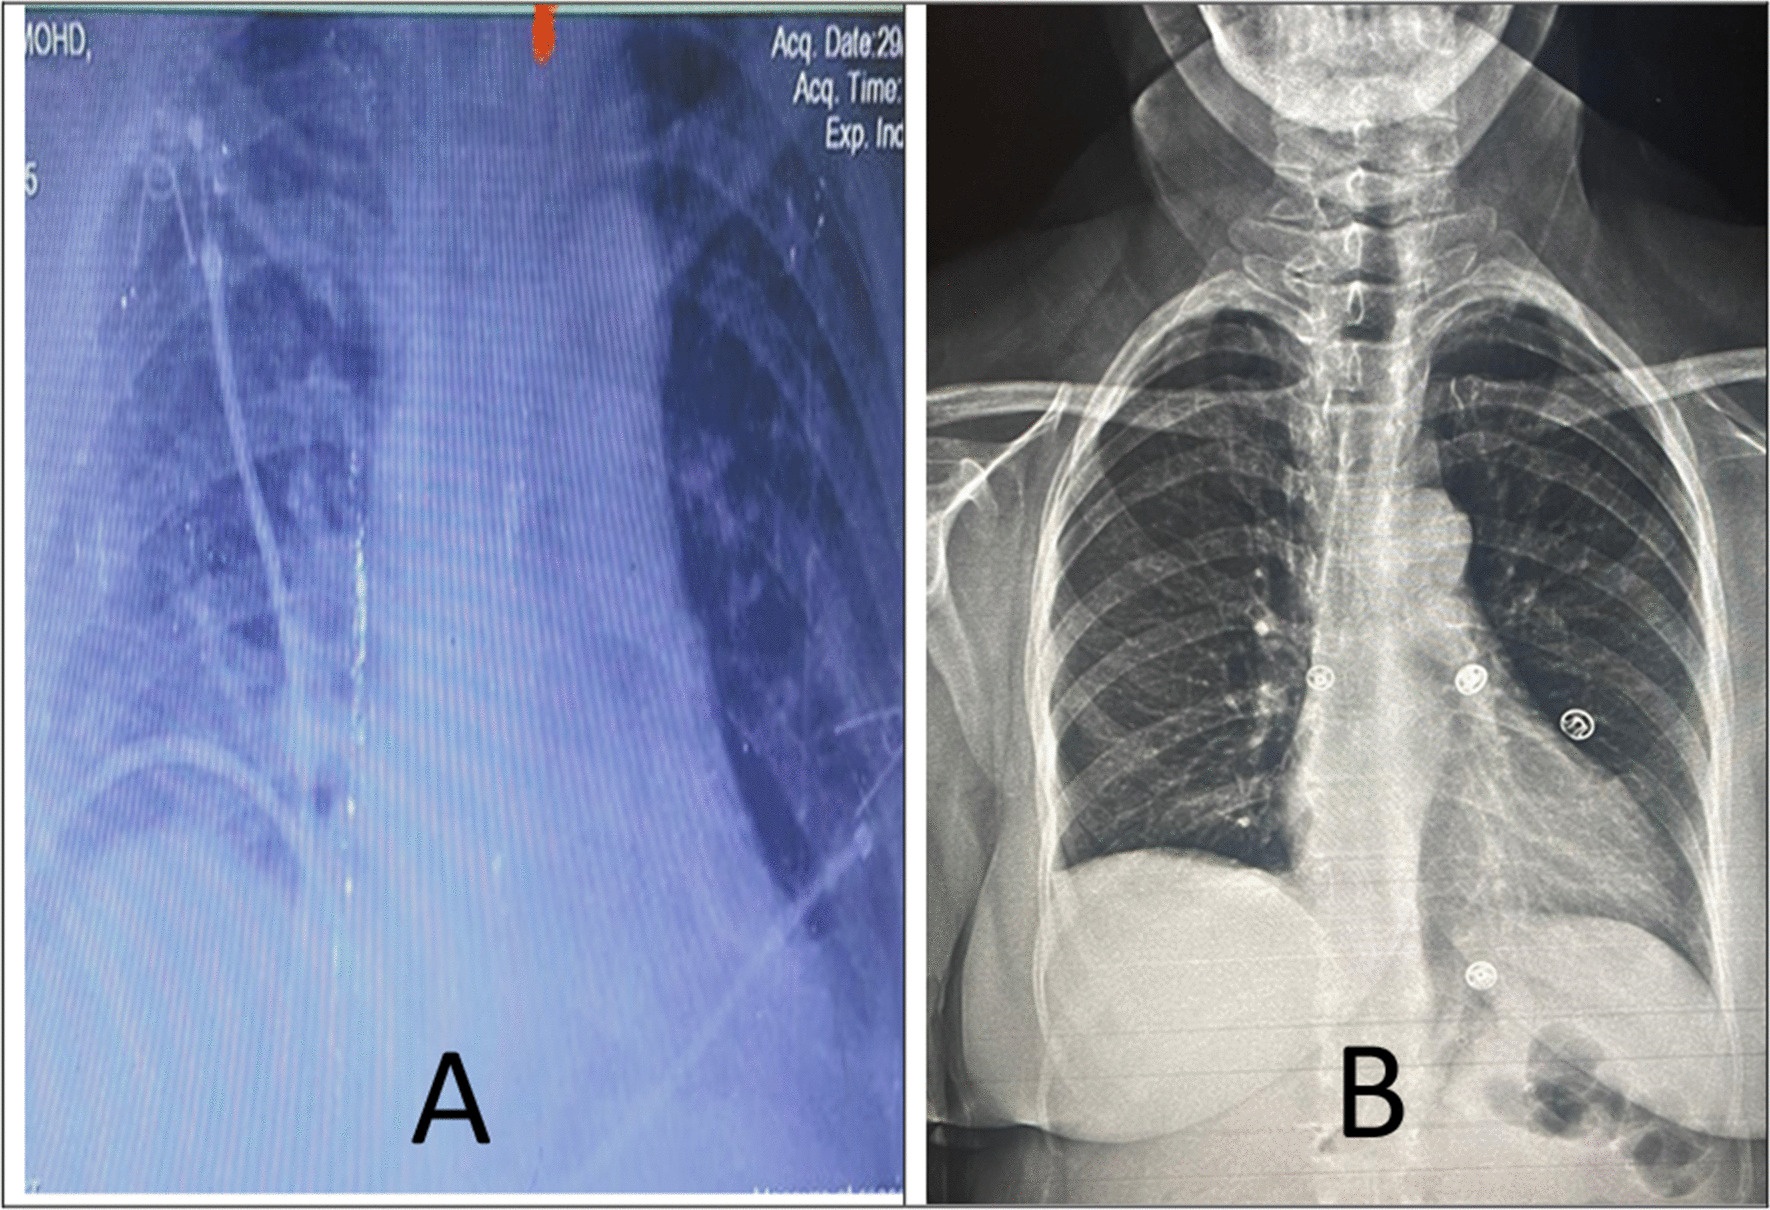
Chest radiography done on the second day postoperation showing white spot infiltration and air under the right diaphragm (A). Chest radiography done after 2 months showed no abnormalities (B)
